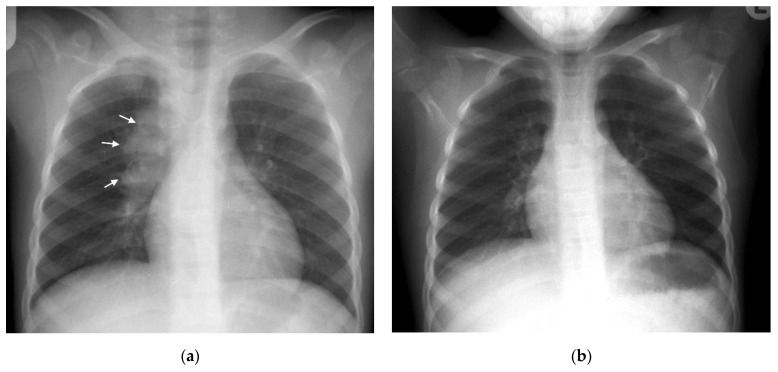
(a,b): Right Paratracheal and Hilar lymphadenopathy before and after treatment. (a) AP chest radiograph of a child at presentation, who later was later confirmed to have pulmonary TB, demonstrates a right-sided lobulated cardio-mediastinal margin with filling of the right hilar point (white arrows) and consistent with right paratracheal and hilar lymphadenopathy. The trachea is displaced to the left, slightly bowed and shows decreased calibre just superior to the carina. There is an oval density seen separately from the scapula in the right lung apex, which in conjunction with the lymphadenopathy, constitutes the Ghon Complex. (b) Post-treatment AP chest radiograph demonstrates complete resolution of the parenchymal focus and lymphadenopathy with a normal right cardio-mediastinal border and return of the trachea to its normal shape and position.
